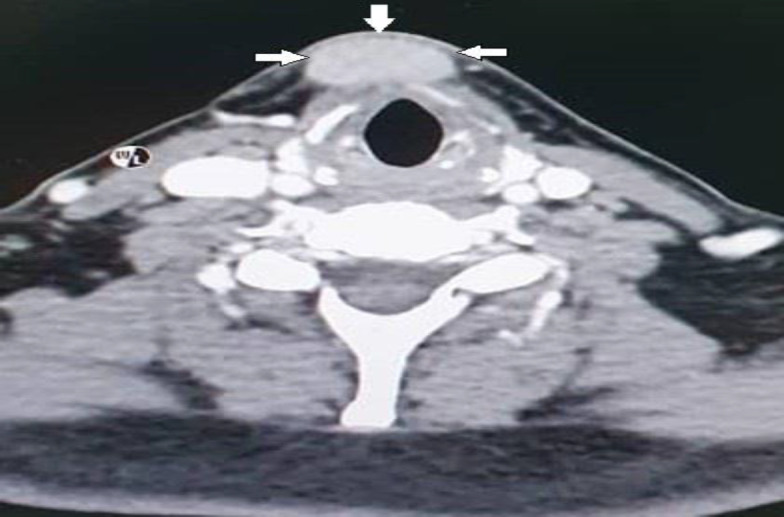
Computed tomographic scan contrast showing homogenously enhancing lesion at midline anterior neck at thyroid cartilage level measuring 1.3 x 2.5 x 2.1cm (white arrows). No clear plane with bilateral strap muscles. Features of erosion of the thyroid cartilage
